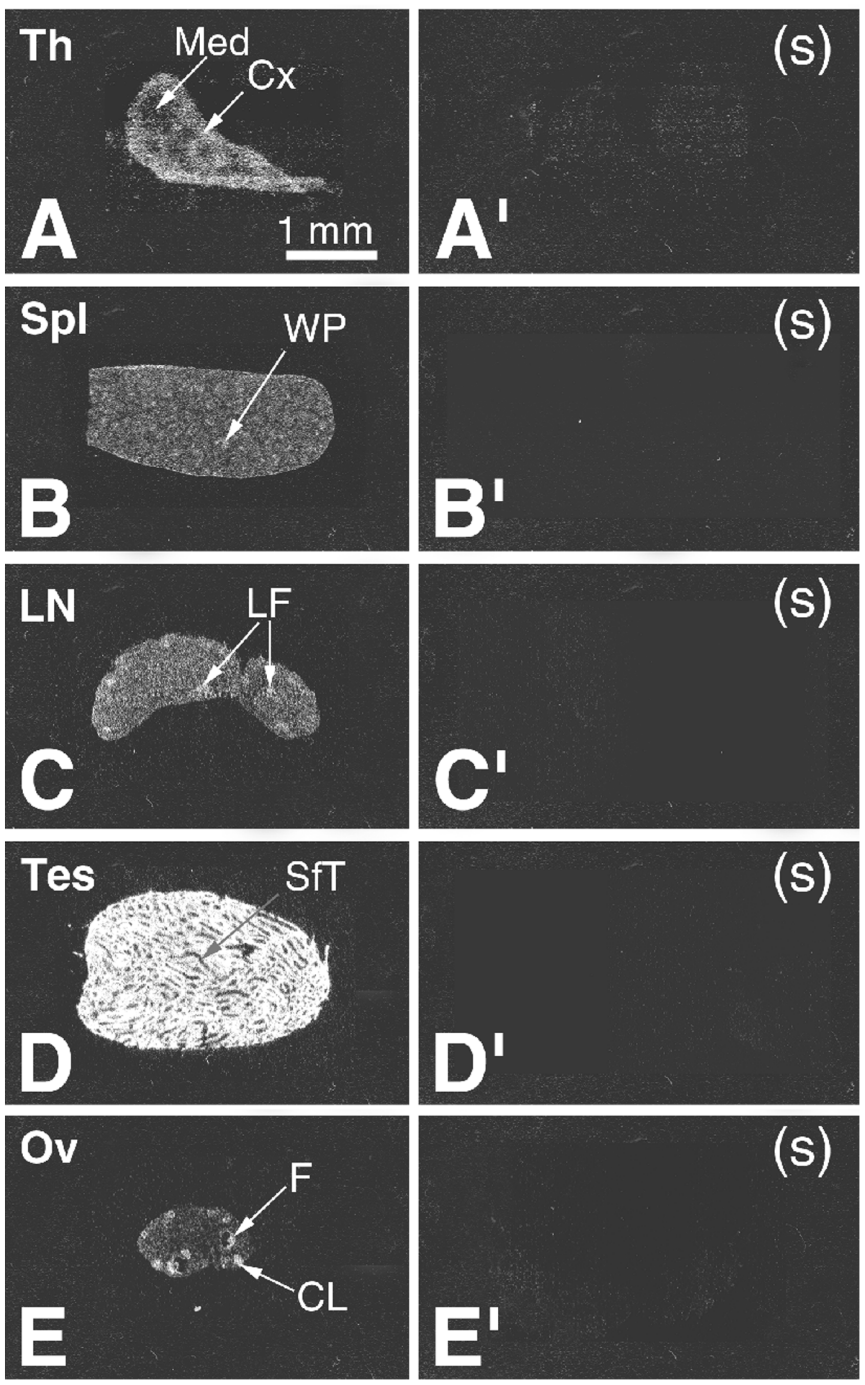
ISH analysis of MLF1-IP expression in individual adult tissues. X-ray autoradiography was under dark-field illumination. The left column shows antisense hybridization, and the right column, sense (control) hybridisation. A and A′: thymus, showing ISH signal mostly in the cortex and less in the medulla. B and B′: spleen, very low-level ISH signal in the white pulp. C and C′: Lymph node, low-level ISH labelling in the lymphatic follicles; D and D′: testis, high-level MLF1-IP expression in seminiferous tubules. E and E′: MLF1-IP mRNA detectable in the follicles and corpus luteum. Original magnification: 10X.
